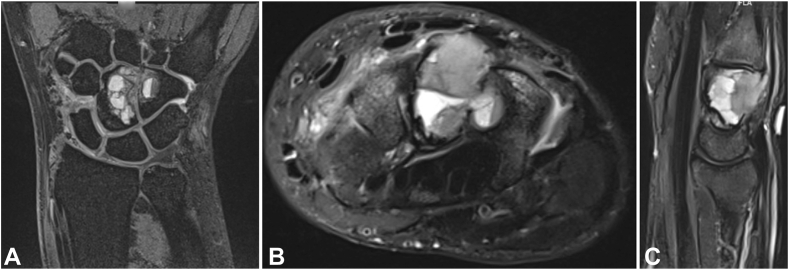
A–C Magnetic resonance imaging coronal and axial T2-weighted and sagittal inversion recovery sections showing a hypointense expansile, destructive lesion originating in the capitate with extension into the hamate.
